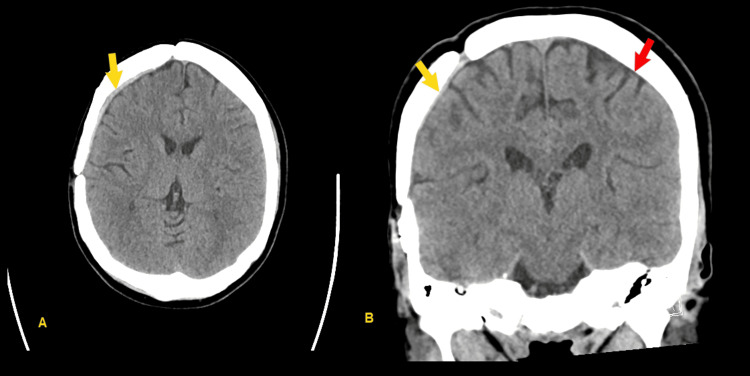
Final CT head, showing near-complete resolution of original subdural hematoma (SDH) (yellow arrows) as well as resolution of second SDH (red arrow) (A) Axial image, (B) Coronal image
